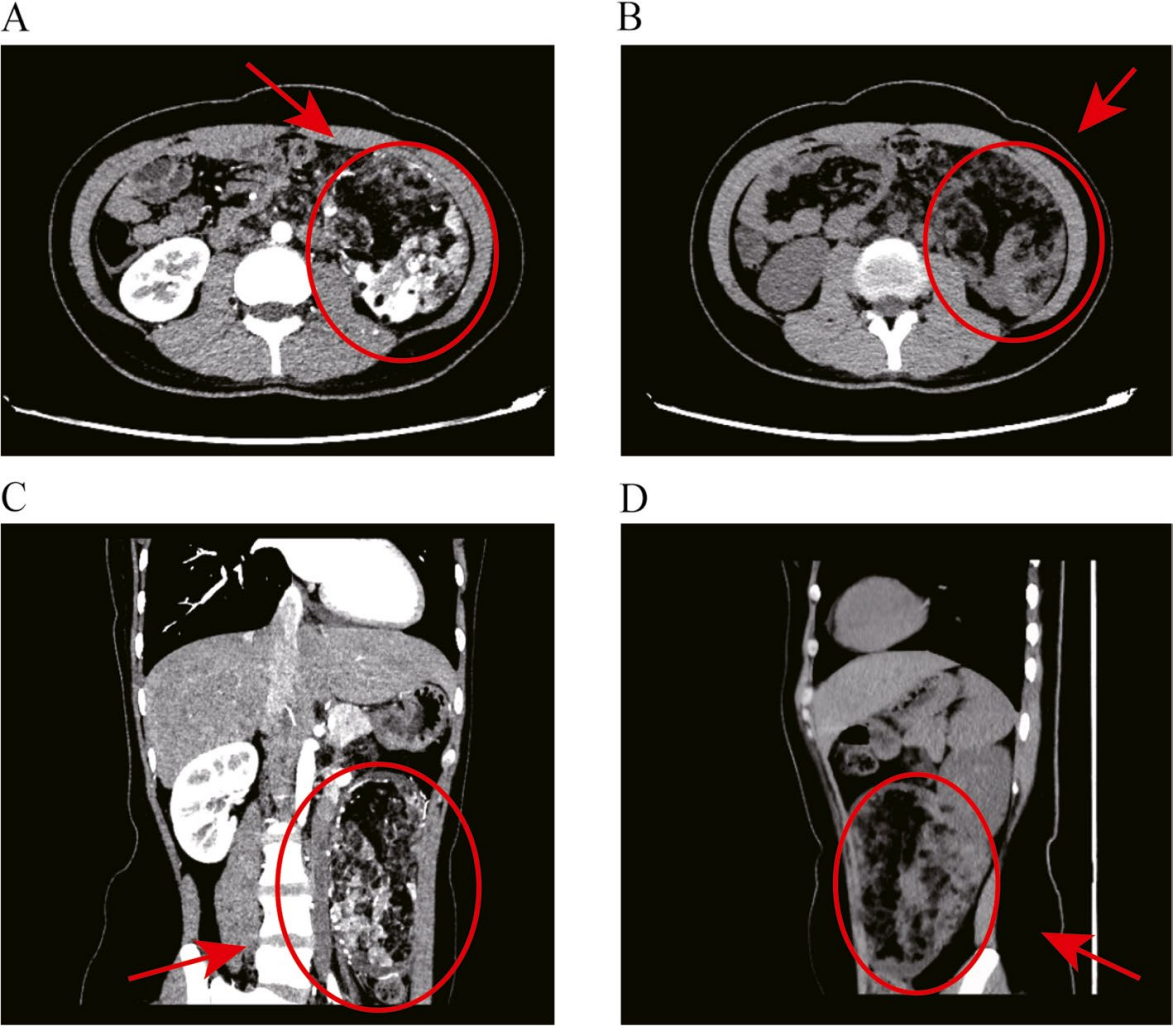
Pre-surgical CT scan. (A: CT Enhancement Scan, Axial section. B: CT Scan, Axial section. C: CT Enhancement Scan, Frontal plane. D: CT Scan, Sagittal plane)
